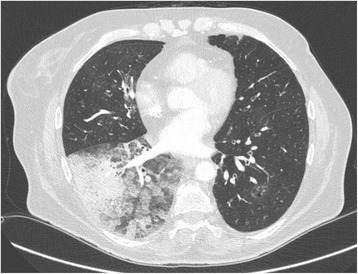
CT-scan showing ground-glass opacities and alveolar consolidation in the right lower lobe
